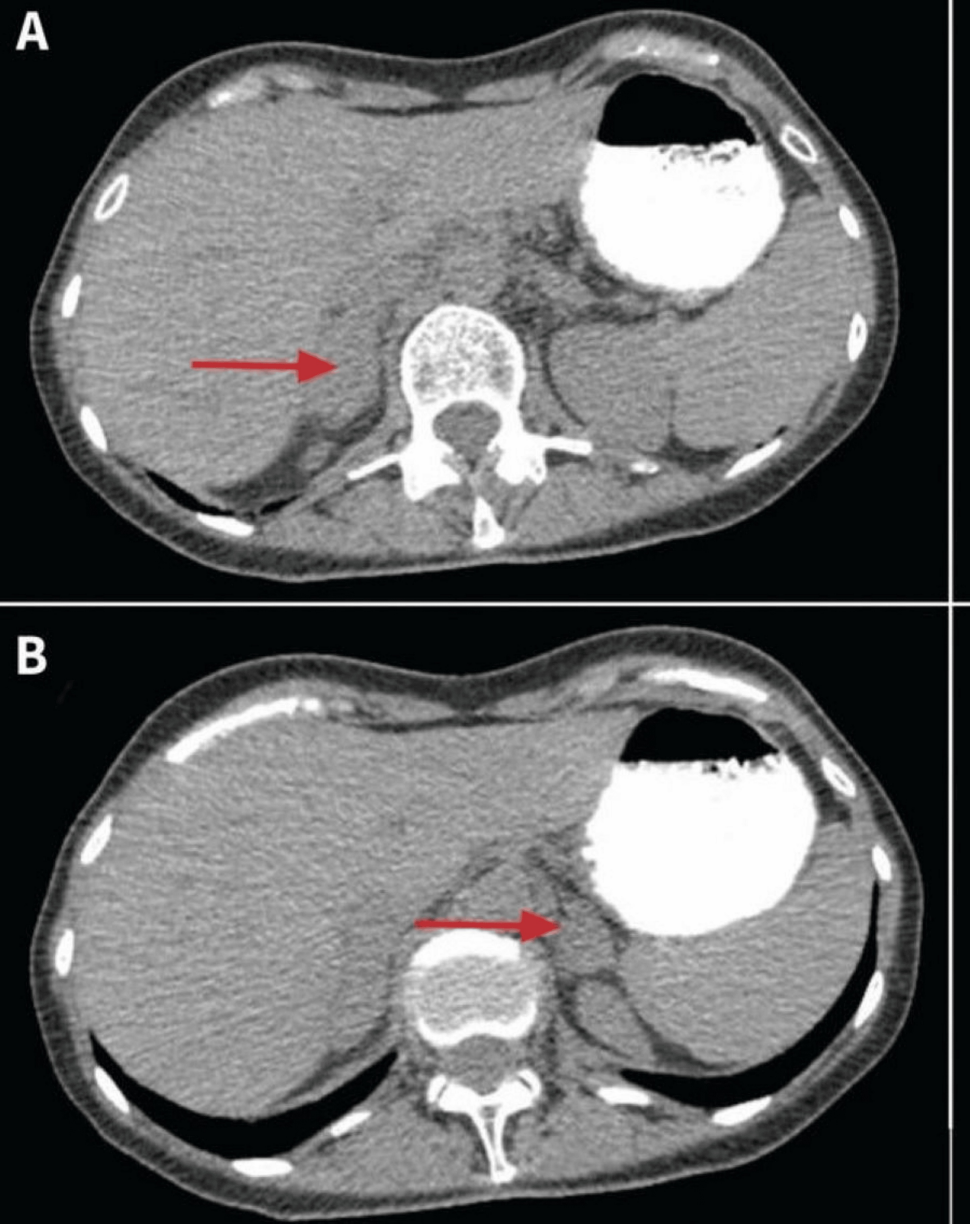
Abdominal CT Scan Showing Bilaterally Atrophic Adrenal Glands Pretreatment
